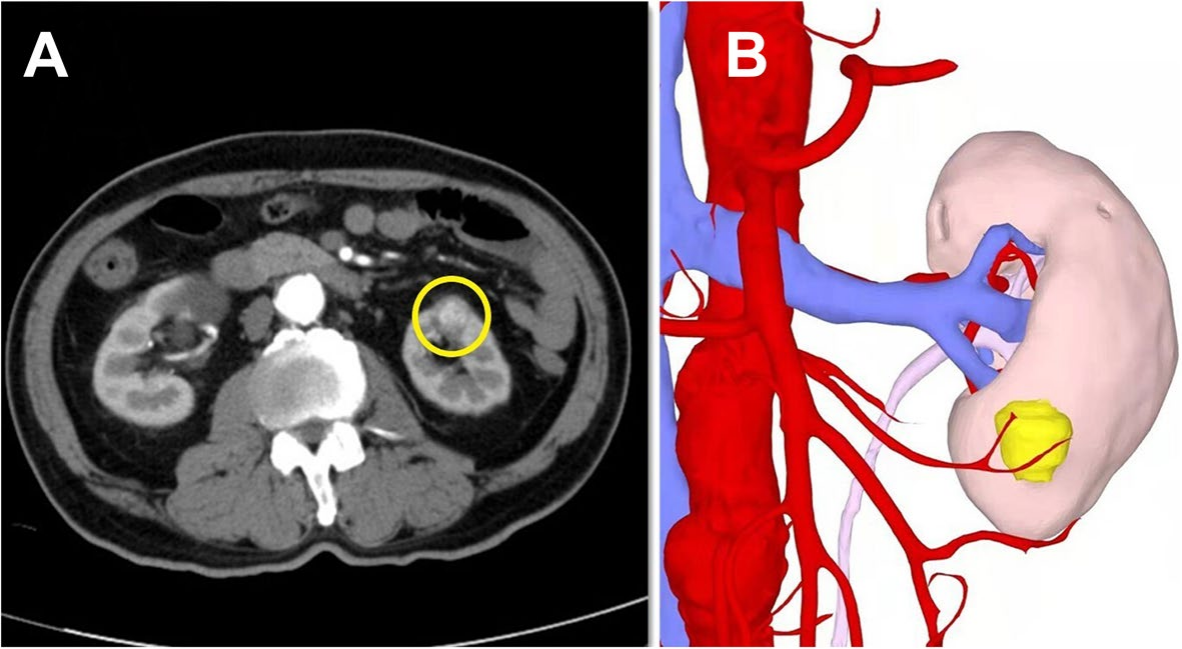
Ventral renal tumor (in yellow). a Computed tomography transverse view. b Three-dimensional reconstruction
